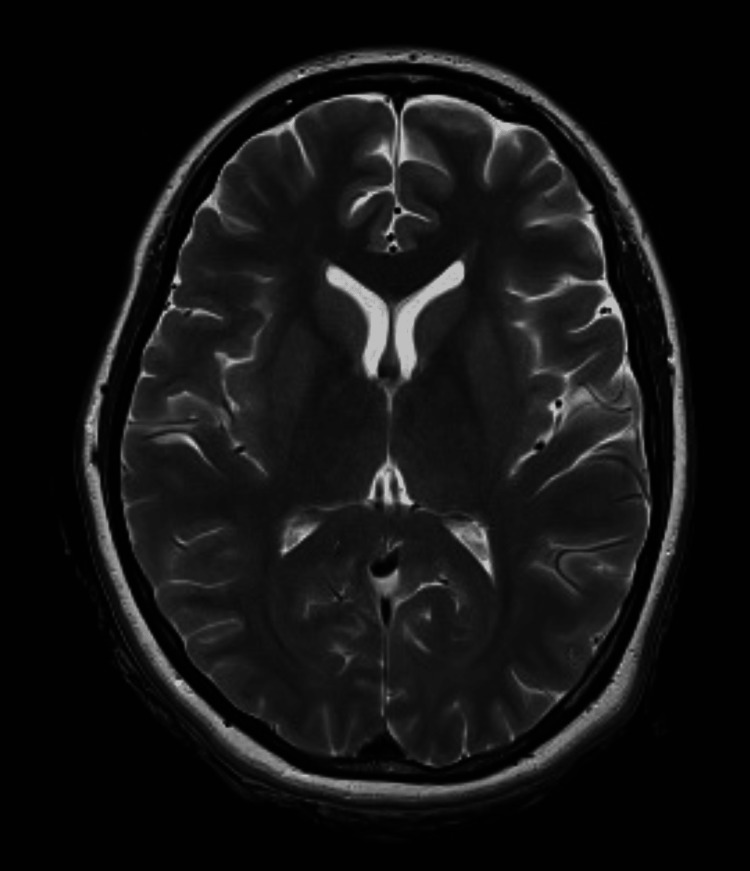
MRI of the brain (axial T2-weighted image) showing normal findings Normal intracranial appearances for age, with no parenchymal signal abnormality or structural lesion MRI: magnetic resonance imaging
